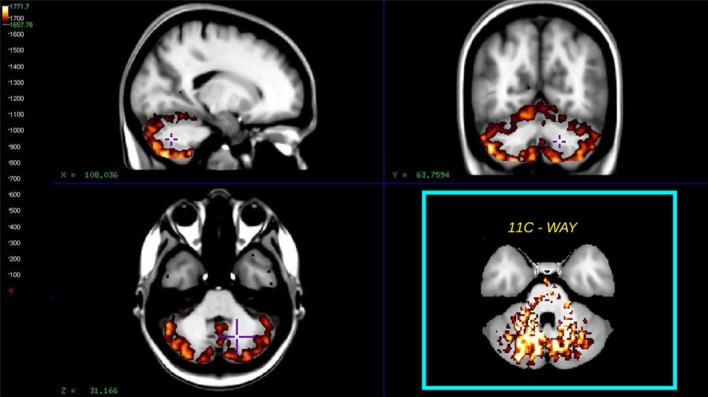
The figure shows the average washout index Θ image calculated over the time interval *T* = 1890 s for all subjects. Mean values >1660 s, superimposed on a non-linear registered average MRI brain, the cyan insert shows the similar reference region for the way compound as found in Møller et al. (2009).
